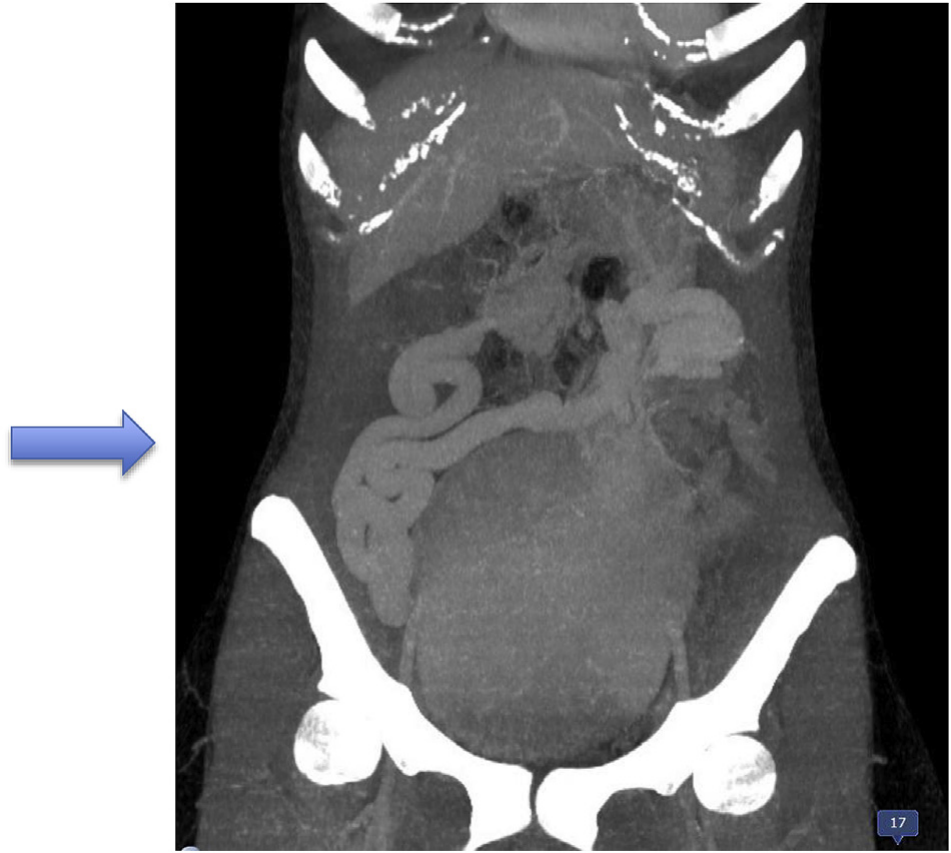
Contrast-enhanced coronal CT image, MPR reconstructions, shows the pelvic location of the megalic spleen and huge ectasic veins.
